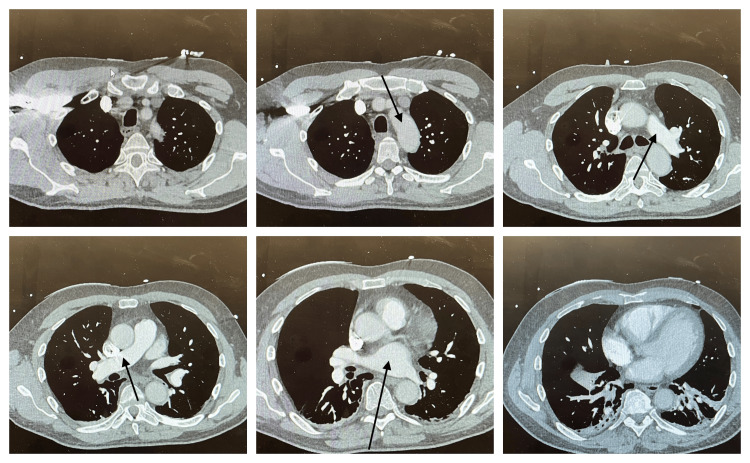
Computed tomography (CT) pulmonary angiogram done to rule out pulmonary embolism (arrows showing no evidence of embolus in the pulmonary arteries)
